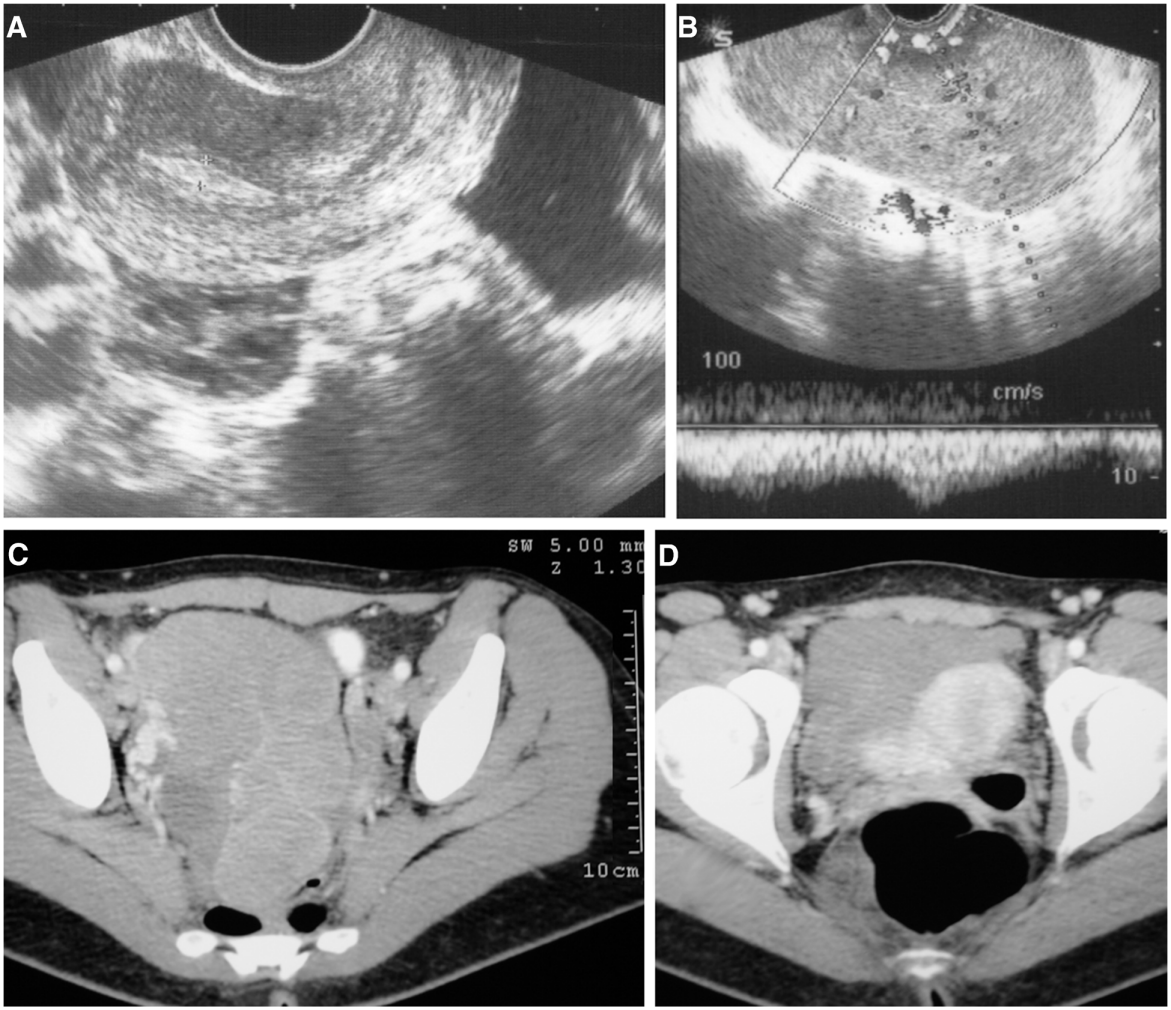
Imaging studies. (A) Uterus and hemoperitoneum transvaginal ultrasound images. (B) Right ovarian tumor and Doppler ultrasonography showing a diffuse vascular mapping with low resistance index. (C) Right ovarian tumor on pelvic computed tomography scan. (D) Tumor and uterus on pelvic computed tomography scan.
